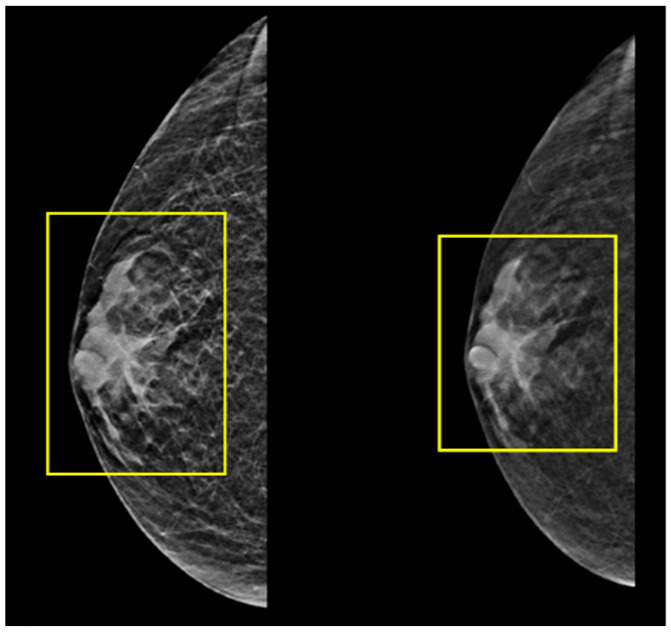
Images from the patient’s ultrasound and mammography screening of the right breast showing prominent breast tissue throughout (yellow squares).
